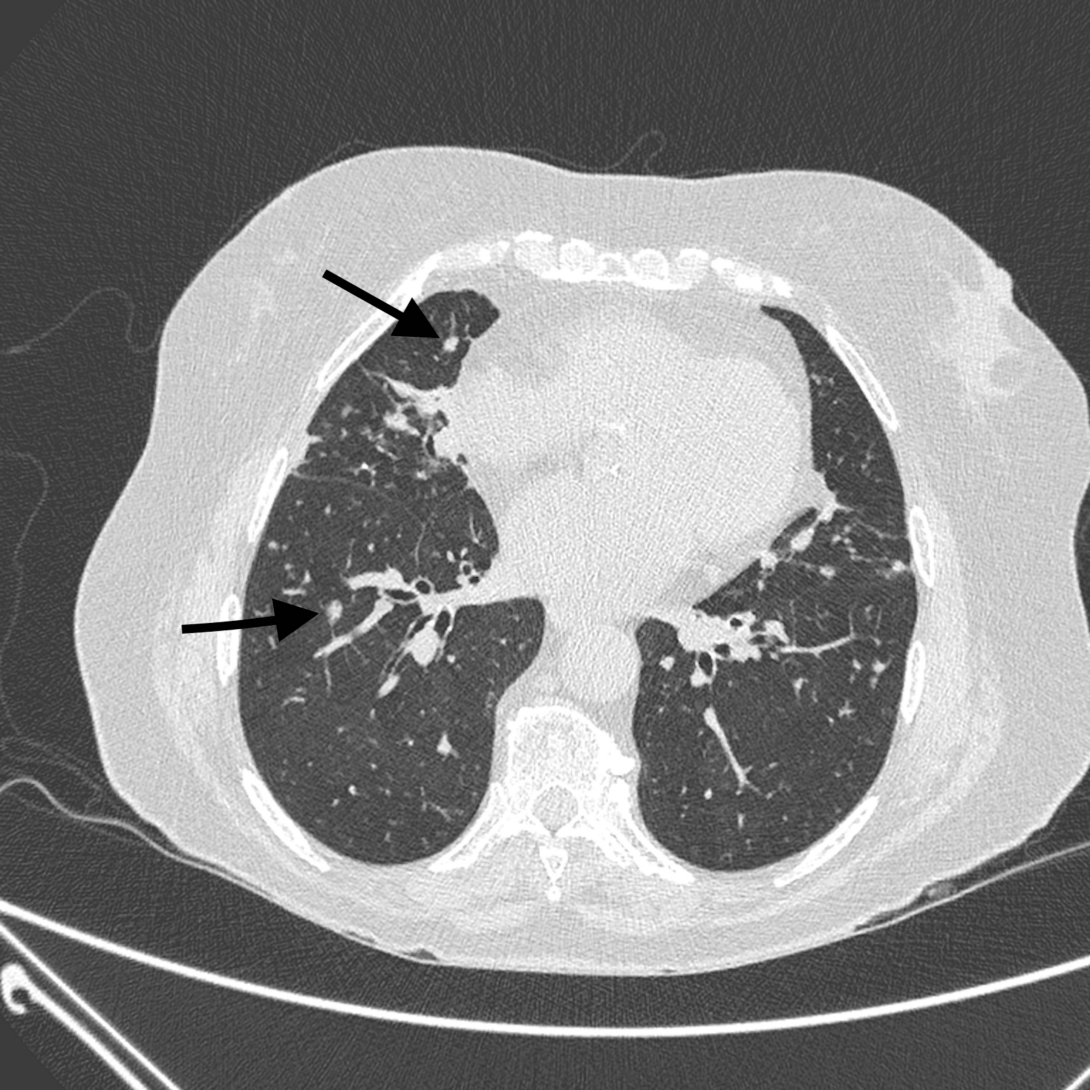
Chest high-resolution computed tomography (HRCT) image at baseline Typical pattern of diffuse idiopathic pulmonary neuroendocrine cell hyperplasia (DIPNECH) with multiple small nodules (black arrows) and areas of mosaic attenuation.
